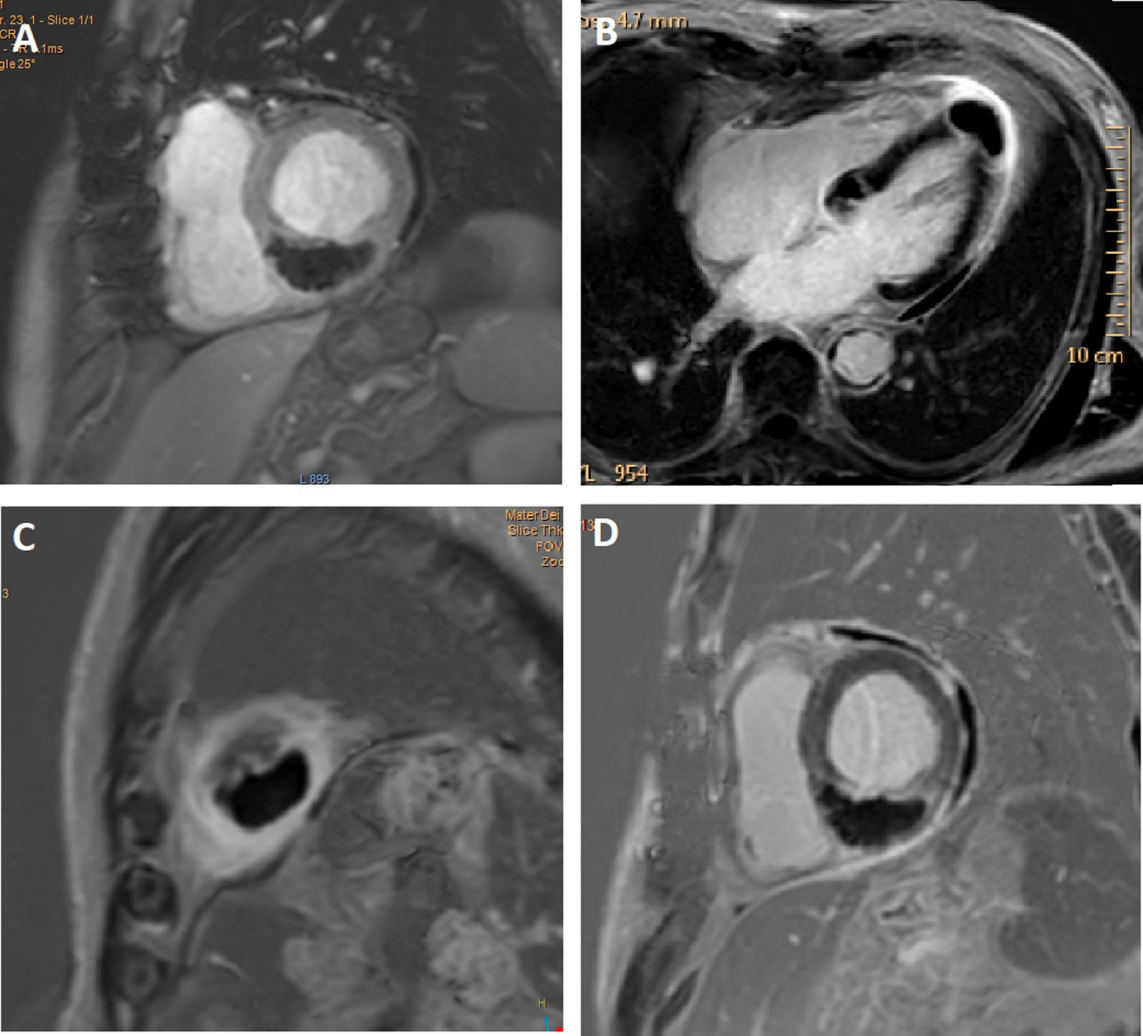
Cardiac Magnetic Resonance Imaging: A Early Gadolinium enhancement in short axis view. Late Gadolinium enhancement CMR images in 4-chamber view (B) and short axis views (C, D) revealing two lesions at base of LV apex and base of interventricular septum. Lesions demonstrate no gadolinium enhancement indicating avascular cores
